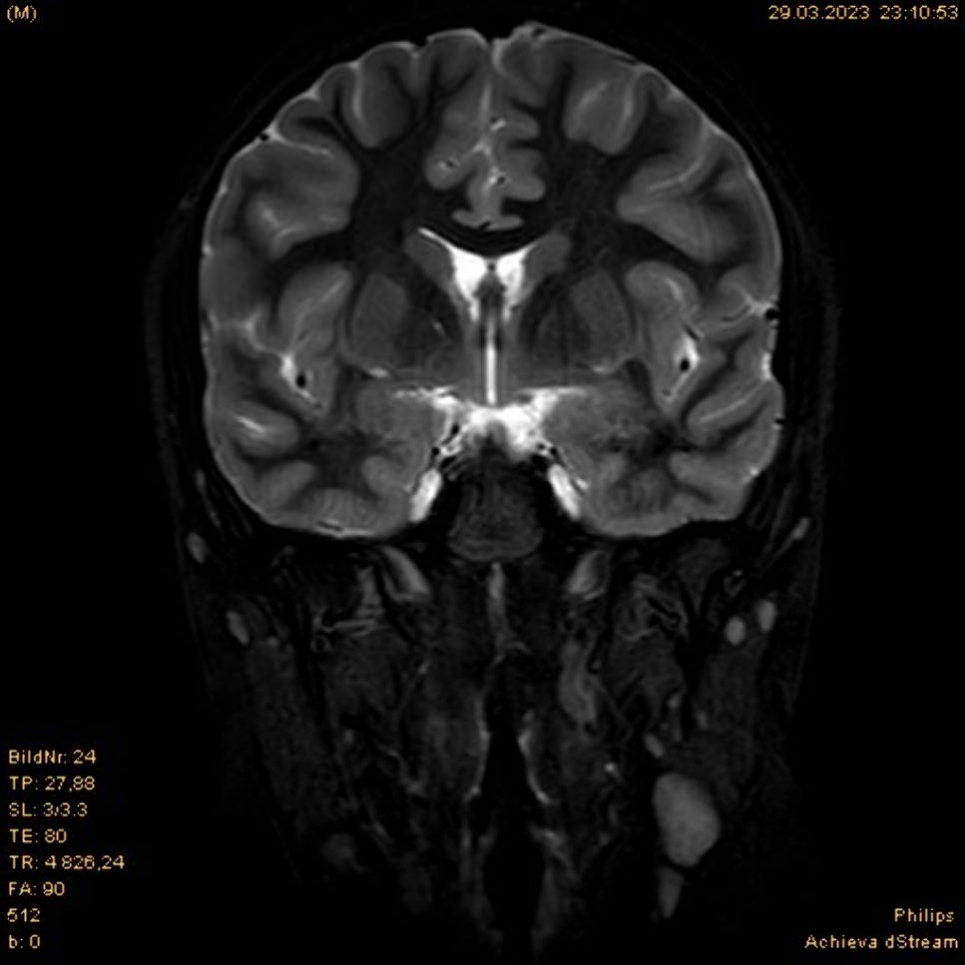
Preoperative cranial MRI in coronal orientation and T2-weighted sequence. Due to the underlying pathology of idiopathic intracranial hypertension, supratentorial ventricular system is very narrow
